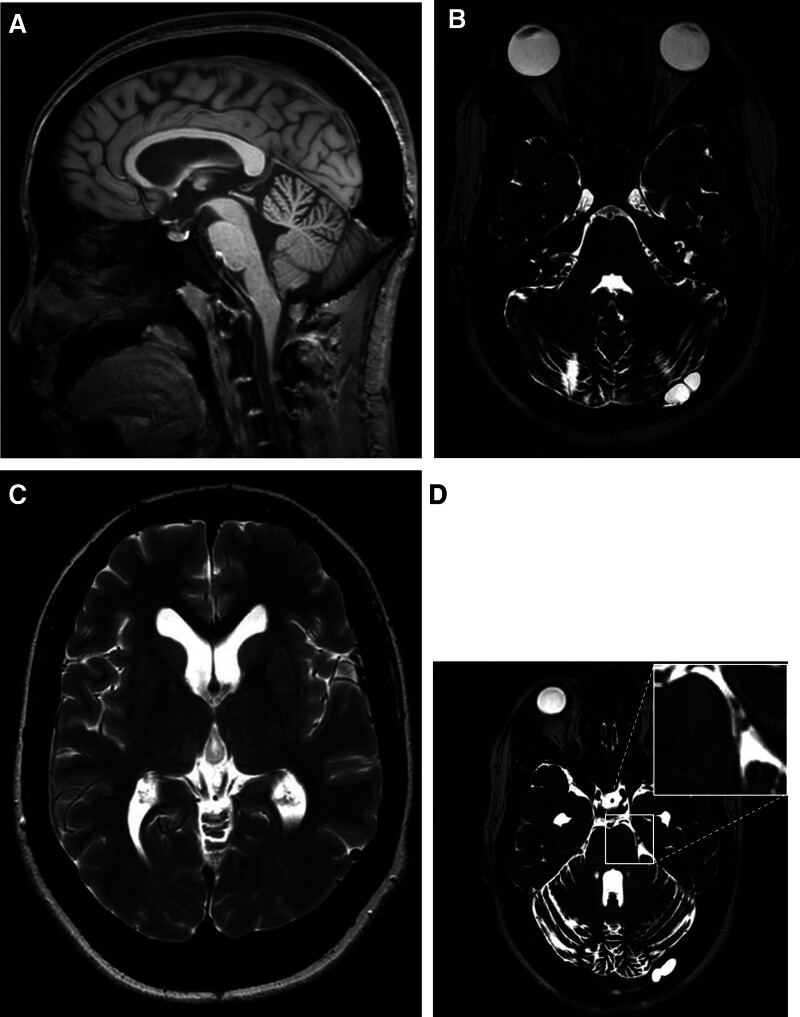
Magnetic resonance imaging following the first-stage decompressive surgery. (A) Sagittal T1-weighted image shows improved brainstem contour and posterior fossa expansion. (B) High-resolution T2-weighted image reveals restoration of the cerebellopontine cisterns. (C) Axial T2-weighted image shows reduced ventricular size, consistent with resolution of hydrocephalus. (D) High-resolution T2-weighted image demonstrates persistent neurovascular compression. The magnified inset highlights the neurovascular conflict at the root entry zone of the trigeminal nerve.
